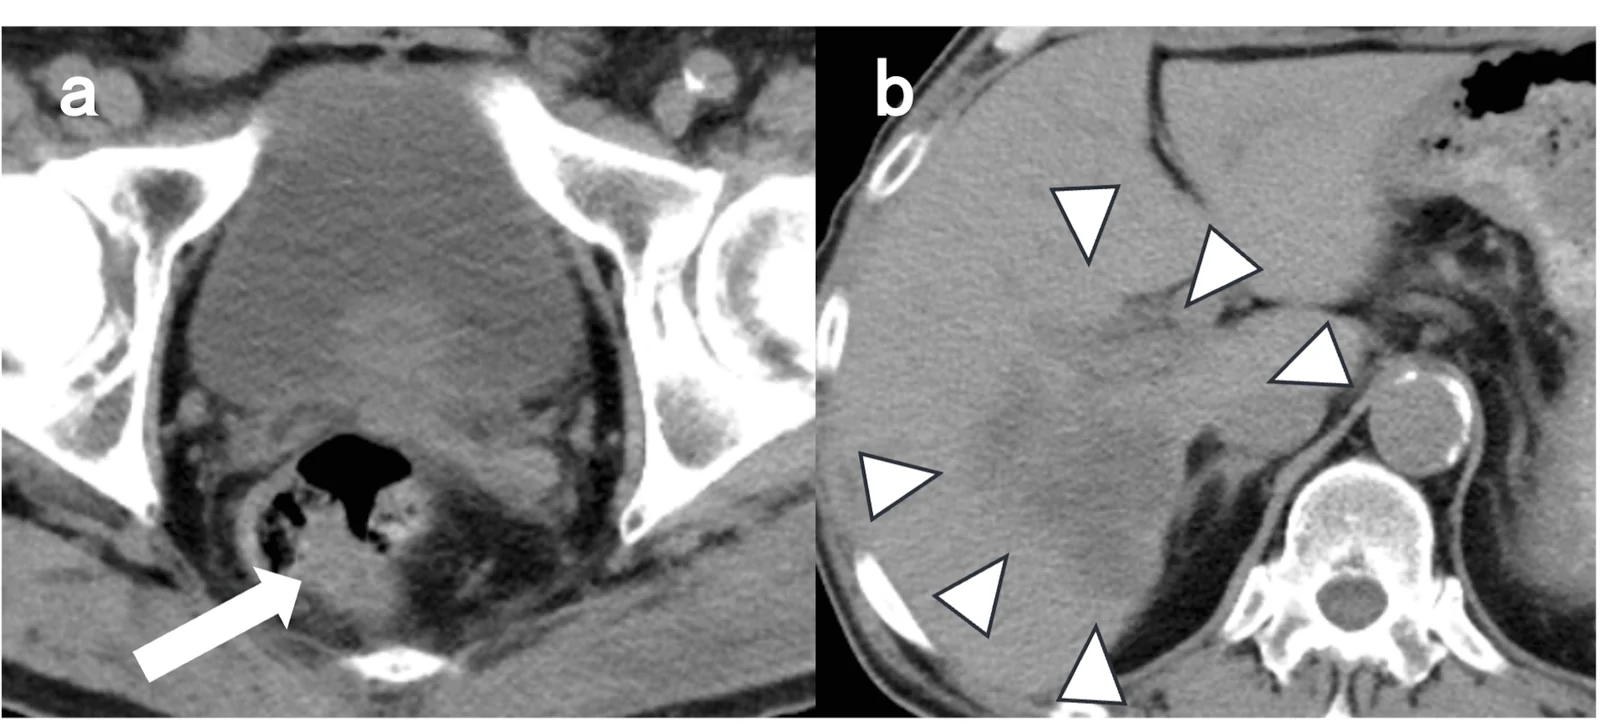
Contrast-enhanced computed tomography images after pembrolizumab treatment (a) Axial contrast-enhanced computed tomography image of the pelvis showing marked regression of the sigmoid lesion, although residual wall thickening with contrast enhancement persisted. The arrow indicates the residual wall thickening of the sigmoid colon. (b) Axial contrast-enhanced computed tomography image of the upper abdomen showing five hypoattenuating lesions in the right hepatic lobe. The dominant lesion, measuring 6.5 cm in maximum diameter, was located in segment 6 near the bifurcation of the anterior and posterior portal venous branches. Additional lesions included a 15-mm lesion near the origin of the right hepatic vein and a 10-mm subcapsular lesion on the surface of segments 5, 6, and 7. All lesions showed uniform hypoattenuation without evident contrast enhancement. Arrowheads indicate the hypoattenuating hepatic lesions.
